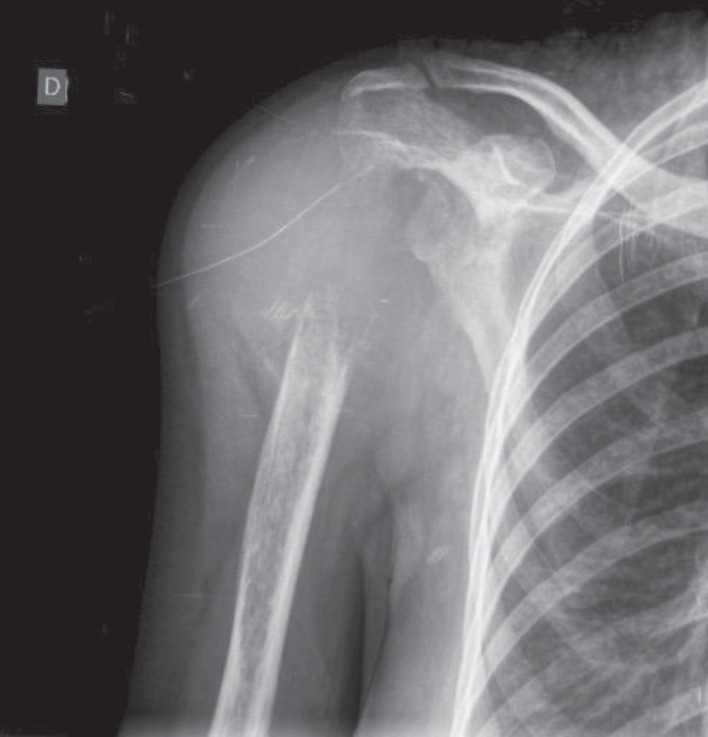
Right arm radiographic. There is a lytic bone lesion on her shoulder with infiltration of local soft tissue. Core biopsies were performed from this site.
